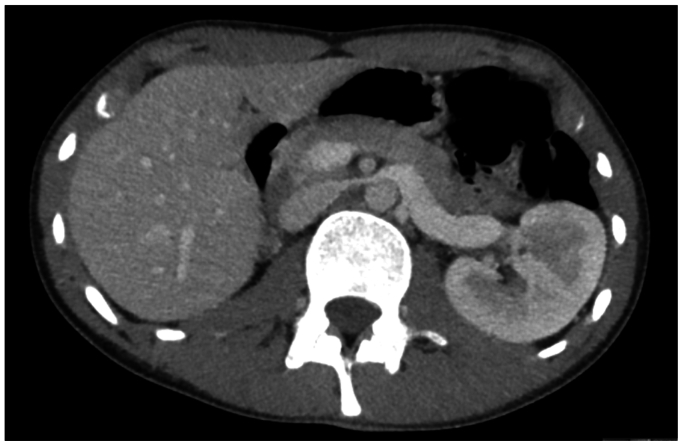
CTA showing compression of the LRV between the superior mesenteric artery (SMA) and the aorta.
